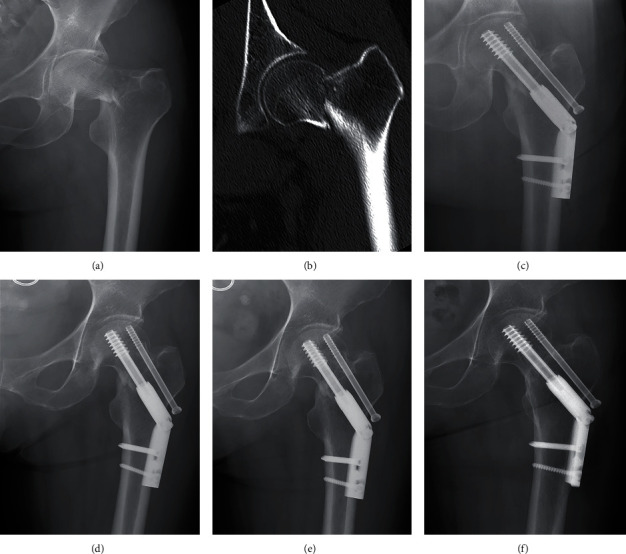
Typical case of positive buttress reduction (female, 52-year-old). (a) Preoperative AP radiograph. (b) CT scan of the same patient. (c) Radiograph immediately after surgery showing positive buttress reduction. (d–f) Radiographs at 1 month, 3 months, and 12 months of follow-up: no complication occurred.
